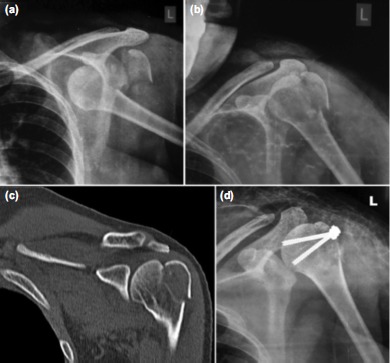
(a) Initial radiograph, (b) Post reduction radiograph, (c) Pre-op CT scan coronal section and (d) Post-op radiograph of left shoulder.
